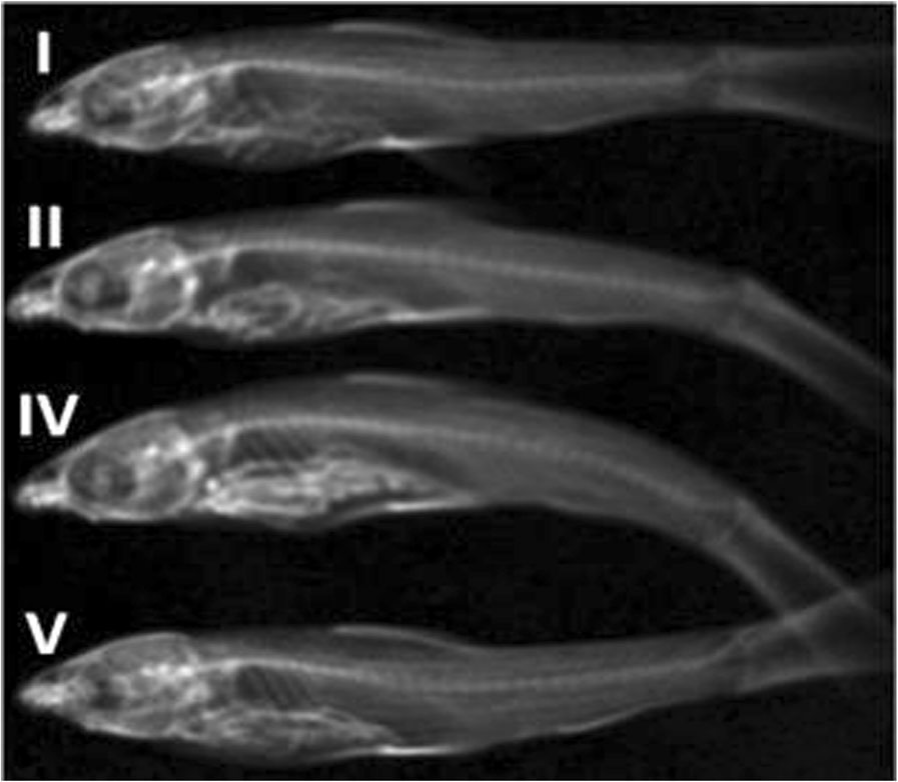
Metal location in fish body obtained by X-ray. Research variant: I—control; II—10 mg/L TiO_2_ in water; III—10 mg/L TiO_2_ in water, without fish; IV—TiO_2_ in plants from variant III; and V—TiO_2_ in the aquarium base from variant III
